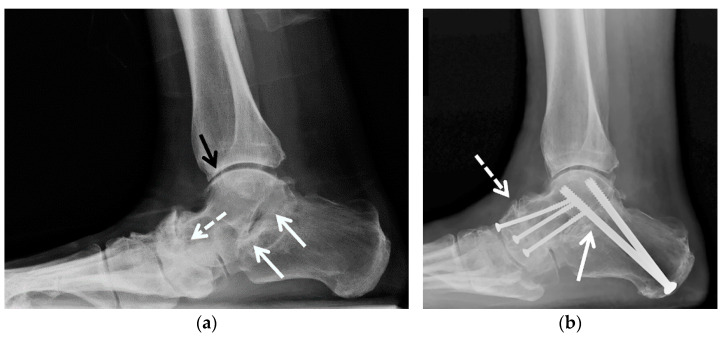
(a,b) A 72-year-old male with pes planus and severe talonavicular greater than subtalar osteoarthritis was treated with double hindfoot arthrodesis. (a) Preoperative weight-bearing lateral radiograph of the right foot shows severe osteoarthritis of the talonavicular (dashed white arrow) greater than subtalar joints (white arrows) and mild osteoarthritis of the tibiotalar joint (black arrow). (b) Weight-bearing lateral radiograph obtained 3 weeks after surgery shows three smaller partially threaded cannulated screws transfixing the talonavicular joint (dashed white arrow) and two larger, partially threaded cannulated screws transfixing the posterior subtalar joint (white arrow). Note the significant improvement of hindfoot alignment.
